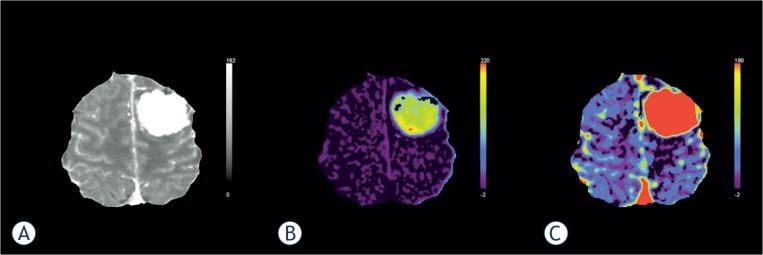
Perfusion computed tomography in brain tumours. Tracer kinetic analysis was performed in a patient with a large tumour in left cerebral hemisphere (A), using Patlak model. The tumour tissue presents with significantly higher permeability values, indicating the immature leaky tumour vessels (B). Unlike the blood volume parametric map (C), permeability imaging also shows some local heterogeneity of tumour tissue.
